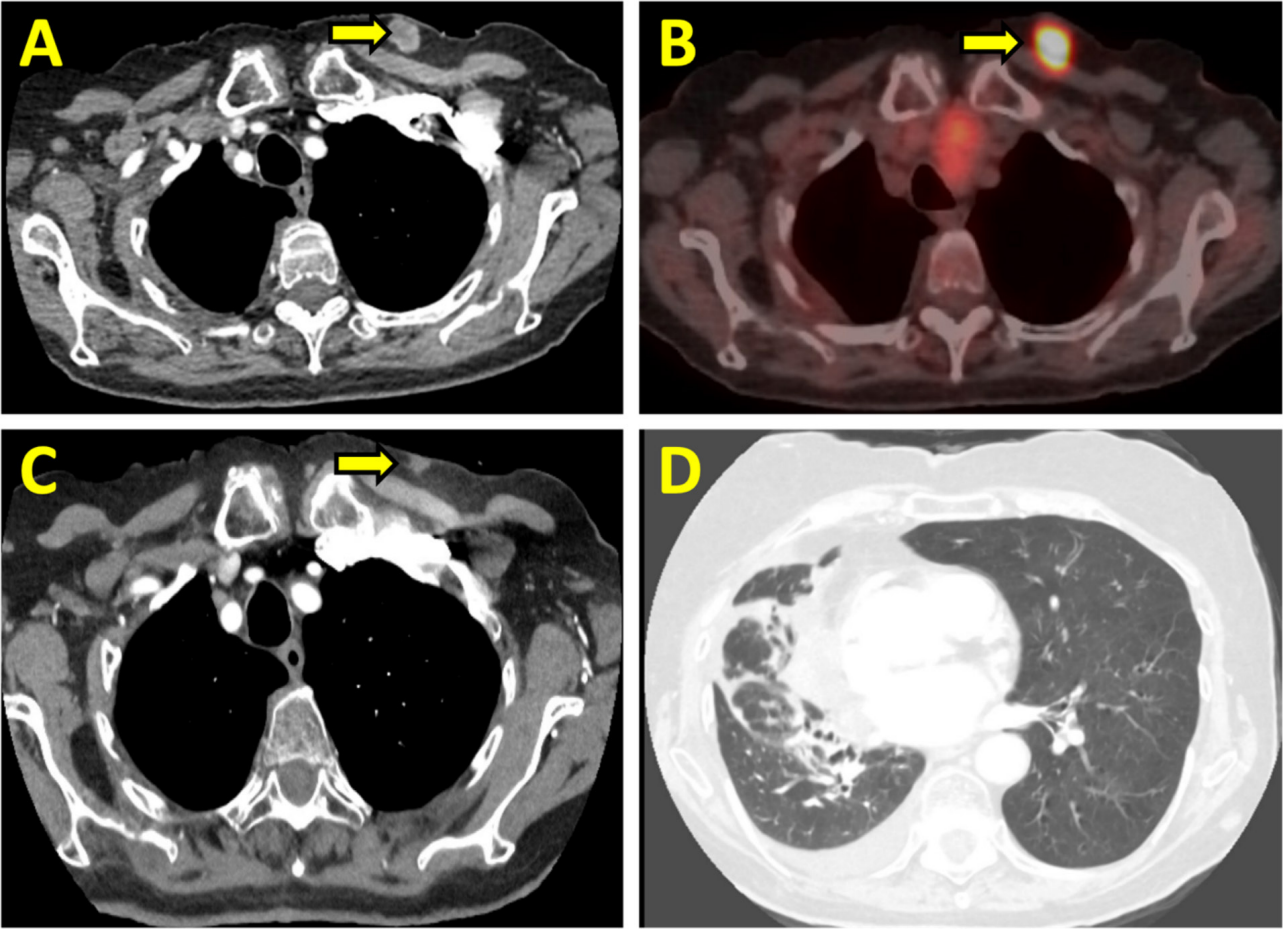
Axial CT chest and PET scan images: (A and B) new subcutaneous nodule in left upper chest wall with FDG uptake (arrows); (C) decrease in size of the subcutaneous nodule post radiotherapy (arrow); (D) progressive regression of right lung mass.
